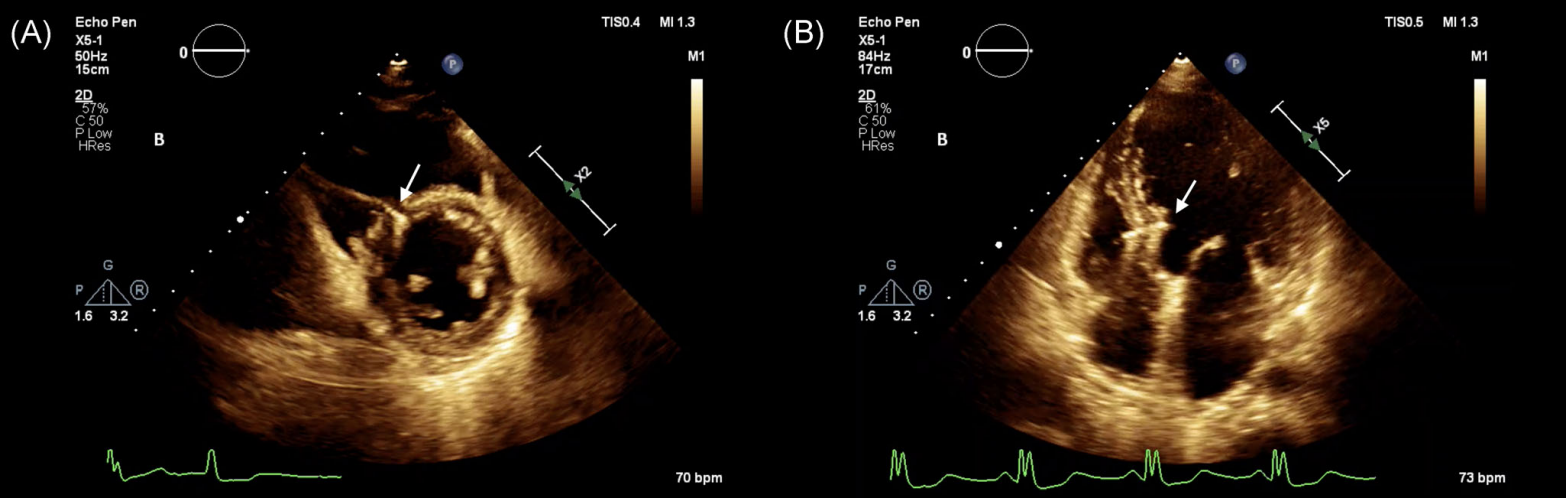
Transventricular septal method for LBBP. A transventricular septal method was applied during the LBBP procedure. Via echo, the tip of active lead was observed to protrude toward the LV cavity while just beneath the endocardium at 3 days after operation. A, Short axis view; B, apical four‐chamber view. LBBP, left bundle branch pacing; LV, left ventricular
